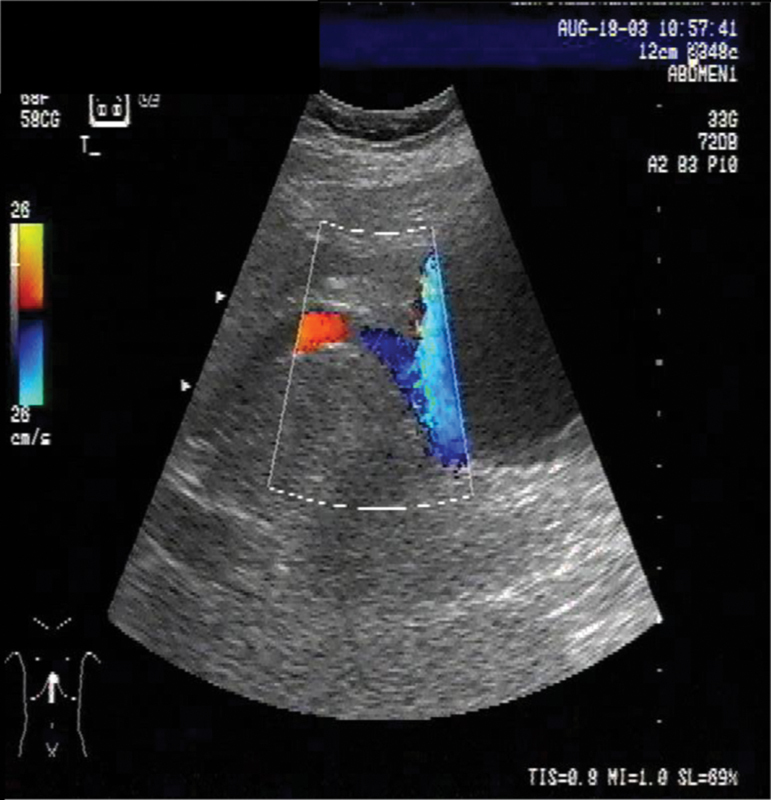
In the power Doppler mode, the superficial abdominal varices are clearly visible.
